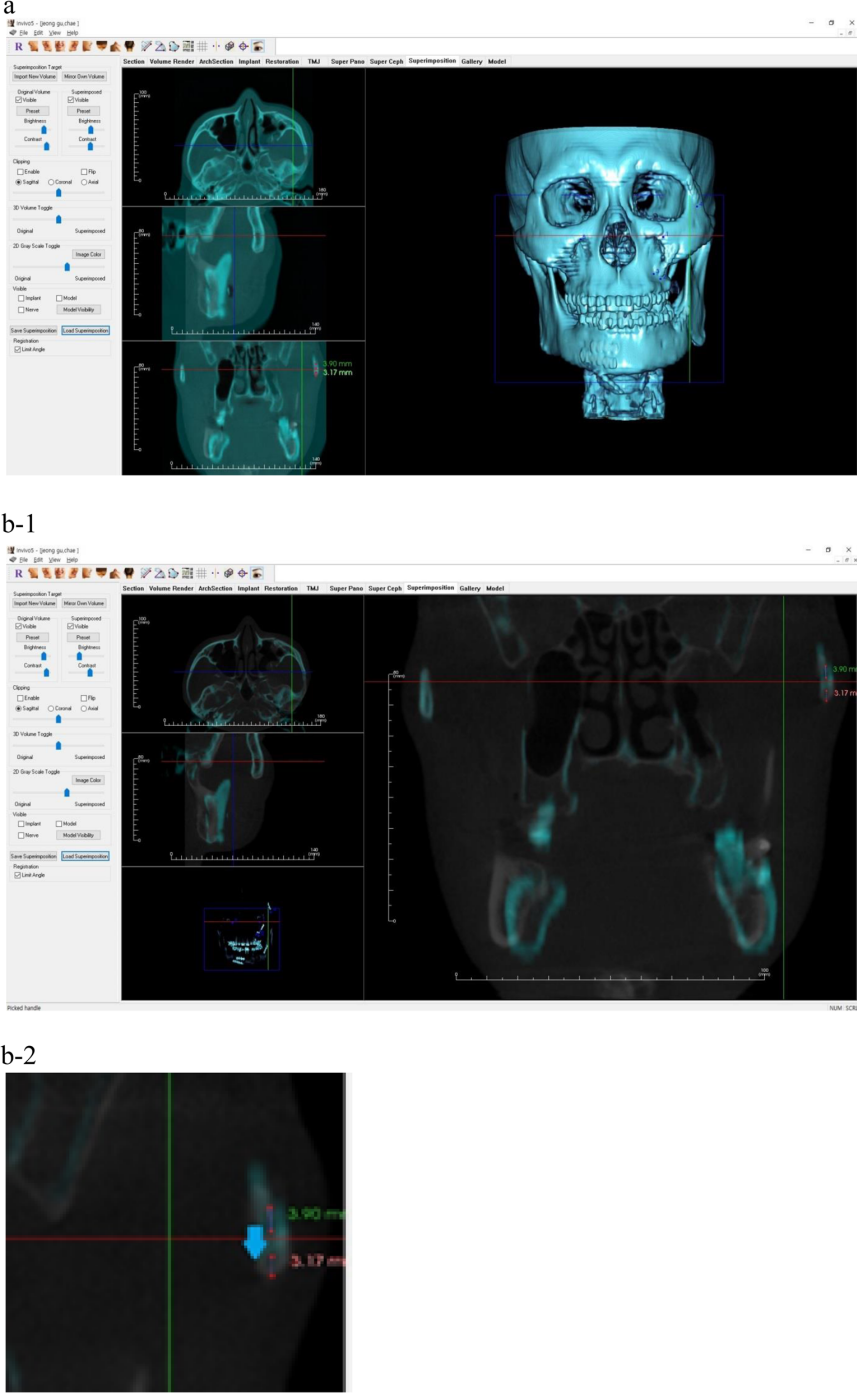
The measurement of amount and direction of displacement of the fractured segment. a CT images are superimposed using Invivo 5, version 5.3 software. As shown in the picture, several landmarks (a total of five in this picture) that do not change after fracture were matched, and the CT image immediately after the operation and the follow-up CT image were superimposed. The blue impression is a postoperative CT image and the white impression is a follow-up CT image. b-1 The measurement of the amount of displacement of the fractured segment. By adjusting the brightness and contrast of the postoperative CT and follow-up CT images, the margins of each bone fragment were well distinguished. After that, the highest points and lowest points of the fracture fragments of the two CT images were connected to each other, and the distance was displayed. b-2 A magnified picture of the fracture site of the figure b-1. The amount of displacement of the fractured segment. The amount of displacement of the fractured segment was calculated by averaging the displacement of the highest points (a green number, 3.90 in this picture) and the lowest points (a red number, 3.17 in this picture) of the fracture. The displacement direction of the fractured segment. The displacement direction of the fractured segment was investigated by the direction of the line connecting the center point of the fracture fragment (blue arrow)
